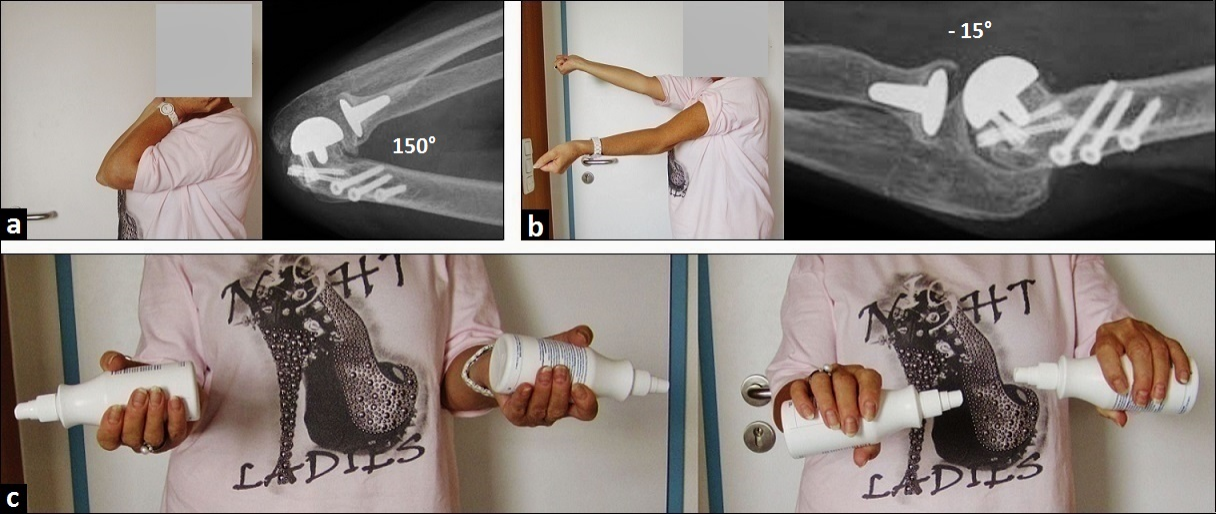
(Case Presentation): (a) Clinical photograph and lateral radiograph demonstrating elbow flexion of 150° of both arms, and there is no impingement between both prosthetic components with terminal range of motion. (b) Clinical photograph and lateral radiograph of elbow extension demonstrating a deficit of 15° to contralateral, and there is no impingement between both prosthetic components with terminal range of motion. (c) Clinical photographs showing each 90° supination and pronation of both forearms.
